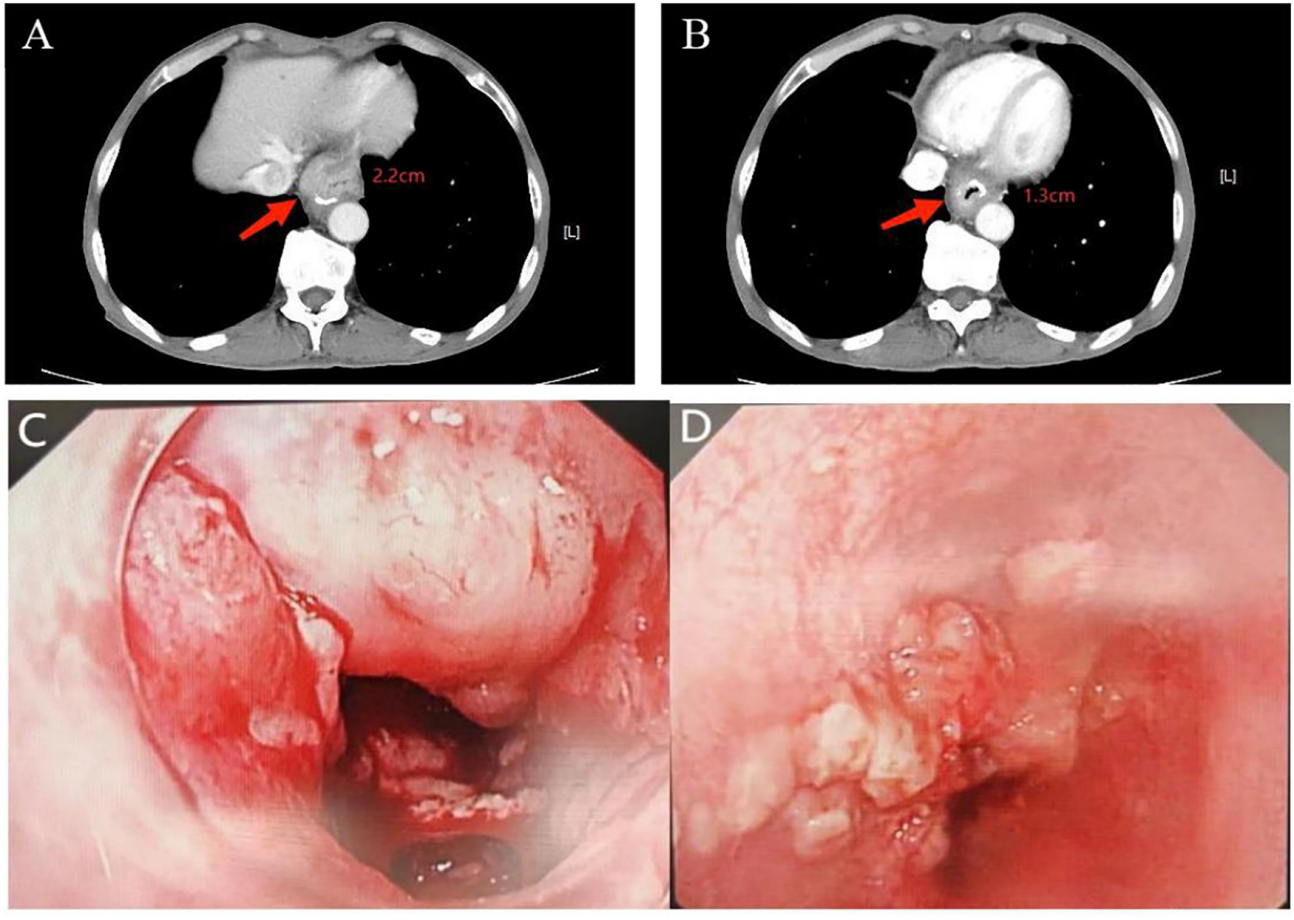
Comparison of cardia lesion thickness on CT and gastroscopy images before and after H101 treatment. (A) Before H101 treatment, the thickest part of the cardia lesion was about 2.2cm; (B) After H101 treatment, the thickest part was significantly reduced to 1.3cm; (C) Before H101 treatment, gastroscopy showed that the cardia lesions were large and protruding into the cavity; (D) After H101 treatment, gastroscopy showed that the cardia lesions were significantly reduced and the local stenosis was significantly improved.
